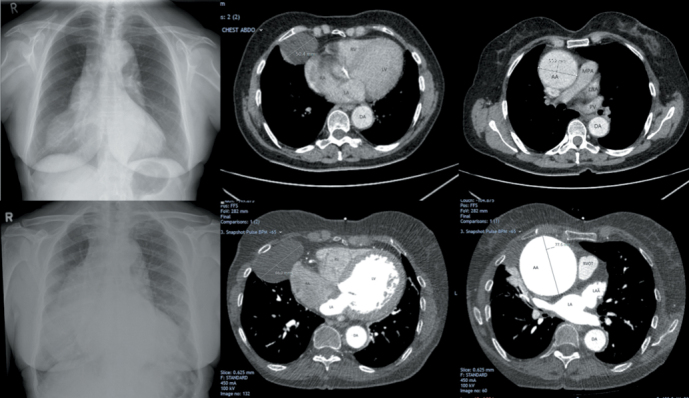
Chest X-ray and CT findings over the course of over 4 years. (A) *CXR March 2015, (B) CT showing pericardial cyst measuring 5 cm along the right heart border,* (C) CT showing ascending aortic aneurysm measuring 5.5 cm at the aortic root*, (D) CXR December 2019, (E) Cardiac gated CT showing pericardial cyst measuring 6.6 cm along the right heart border, and (F) cardiac gated CT showing giant ascending aortic aneurysm measuring 7.7 cm. Abbreviations: AA, ascending aorta; DA, descending aorta; LA, left atrium; LAA, left atrial appendage; LV, left ventricle; MPA, main pulmonary artery; PC, pericardial cyst; PV, pulmonary vein; RA, right atrium; RV, right ventricle; RVOT, right ventricle outflow tract*
